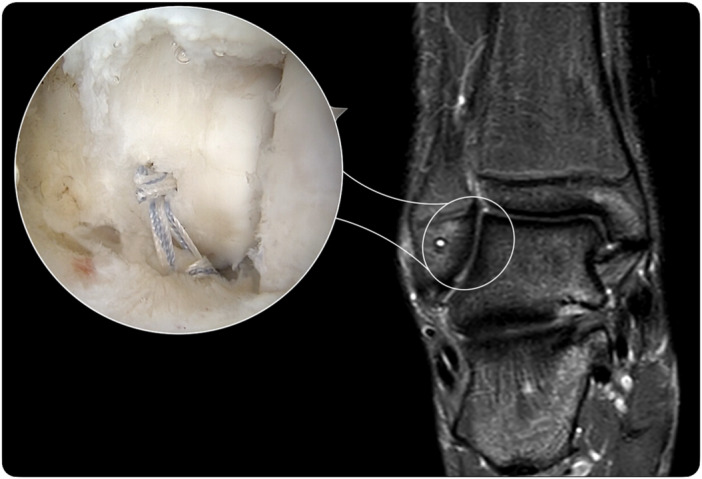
Postoperative MRI (right) and corresponding arthroscopic view (left) showing the safe distance between the fibular physis and the knotless anchor. MRI, magnetic resonance imaging
